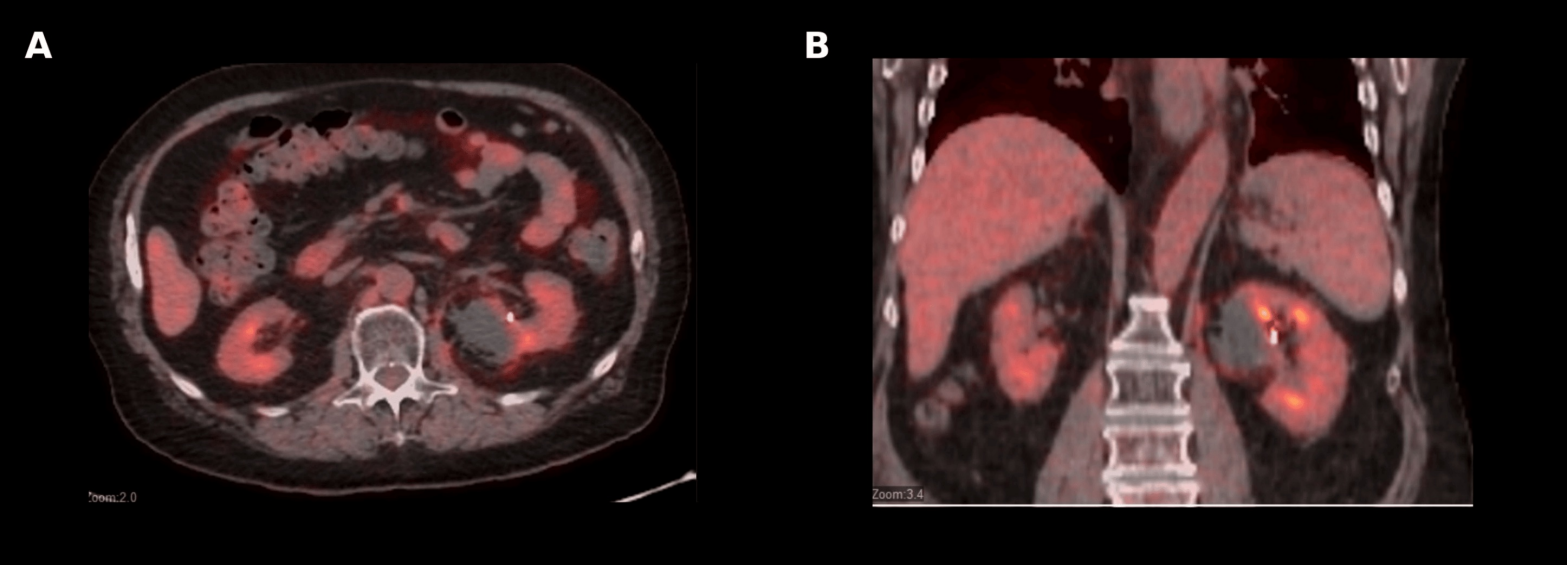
Four-week post-ablation PET/CT demonstrating interval metabolic response (A) Axial positron emission tomography/computed tomography (PET/CT) image obtained four weeks after ablation showing no abnormal fluorodeoxyglucose (FDG) uptake at the ablation site in the left kidney; (B) Coronal PET/CT image demonstrating an absence of residual or recurrent metabolic activity within the treated region, consistent with a favorable post-treatment response.
